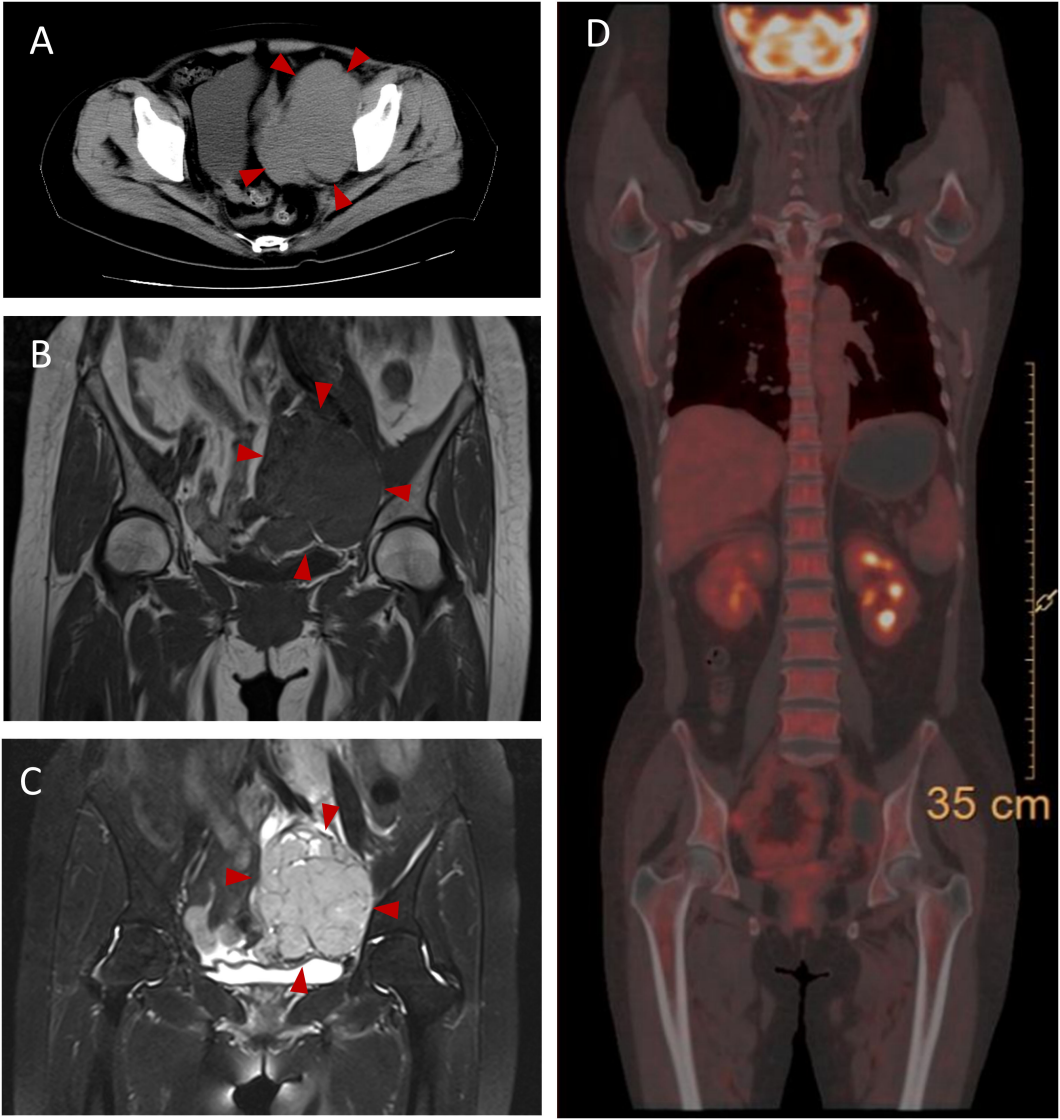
Abdominal CT and MRI and PET-CT findings in this case. (A) Abdominal CT shows an equal-denstiy mass pointed by the red arrowheads in the left lower abdomen. (B) T1-weighted image shows a hypointense mass pointed by the red arrowheads in the left lower abdomen. (C) T2-weighted image shows a hyperintense mass pointed by the red arrowheads in the left lower abdomen. (D) PET-CT shows no neoplasm indications. CT, computer tomography; MRI, magnetic resonance imaging; PET, positron emission tomography.
